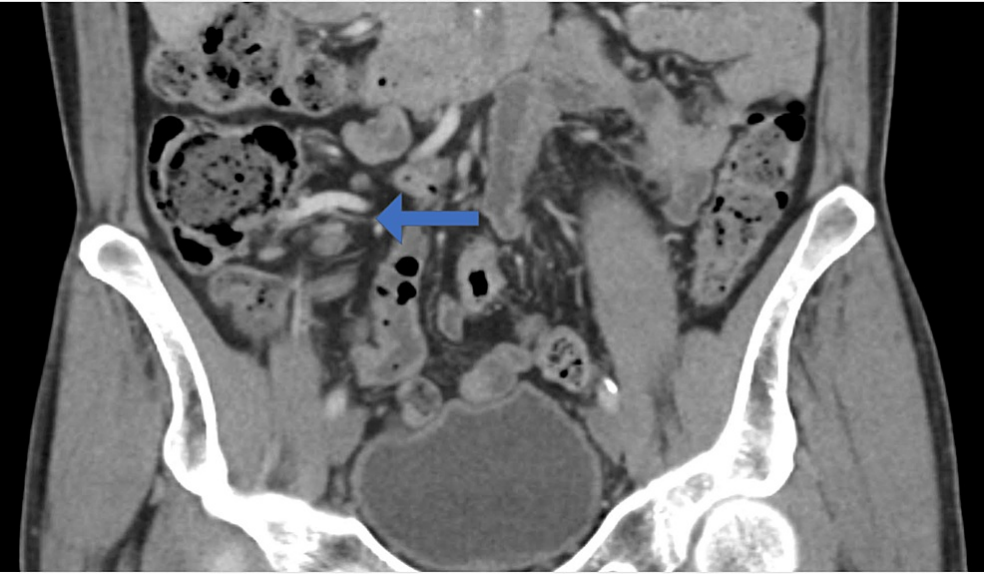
Coronal computed tomography scan done on June 12th.
